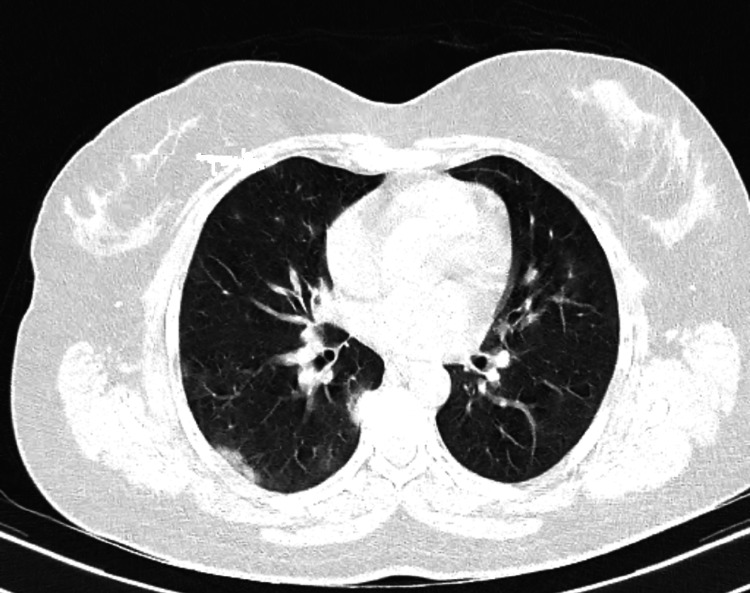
Axial CT section with lung window.
